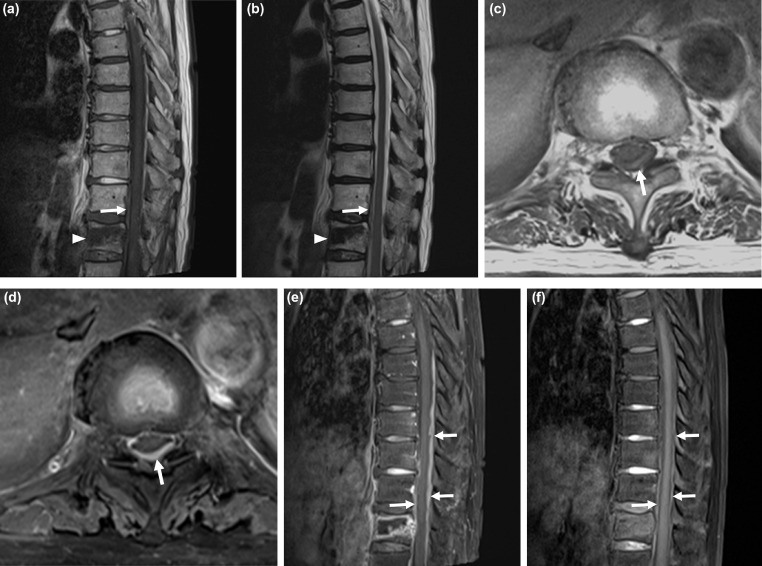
An 89-year-old woman with acute compression fracture and associated acute spinal subdural hematoma. (A and B) The sagittal T1-weighted (A) and T2-weighted (B) images show iso- to slight hyperintensity and hyperintensity, respectively, of the subdural hematoma. The acute subdural hematoma is indistinct from the cerebrospinal fluid (CSF) on both images (arrow). Acute T12 compression fracture is also seen (arrowhead). (C) The axial T1-weighted image confirming the subdural location of the hematoma (arrow). (D and E) The axial (D) and sagittal (E) fat-saturated enhanced T1-weighted images show hyperintensity of the hematoma (arrows). (F) The sagittal non-enhanced fat-saturated T1-weighted image taken 3 days later shows a slight decrease in size of the subdural hematoma. (arrows).
